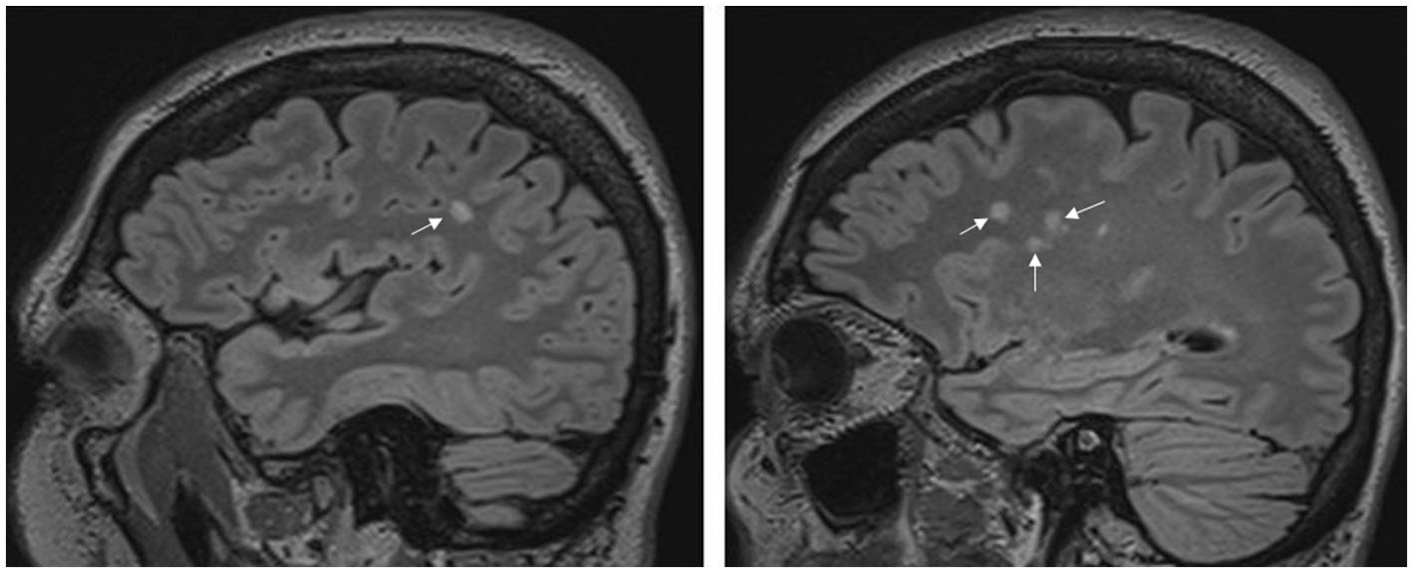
Migraine patient with intrahemispheric white matter lesions. The sagittal fluid-attenuated inversion recovery (FLAIR) images belong to one patient and show high signal intensities in the white matter. The arrows show the lesions.
